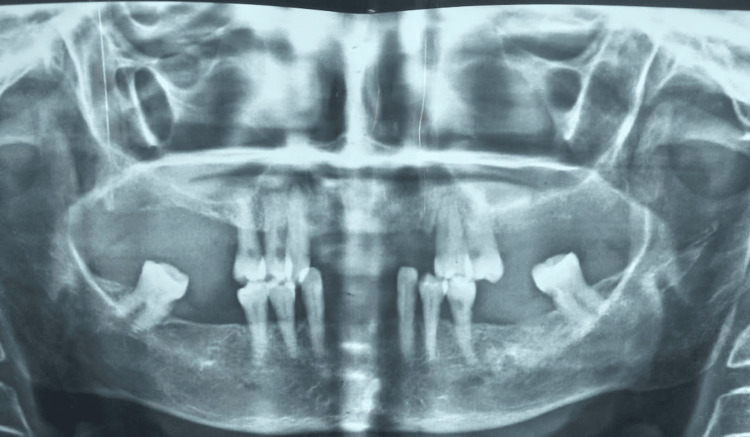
Orthopantomograph image.
